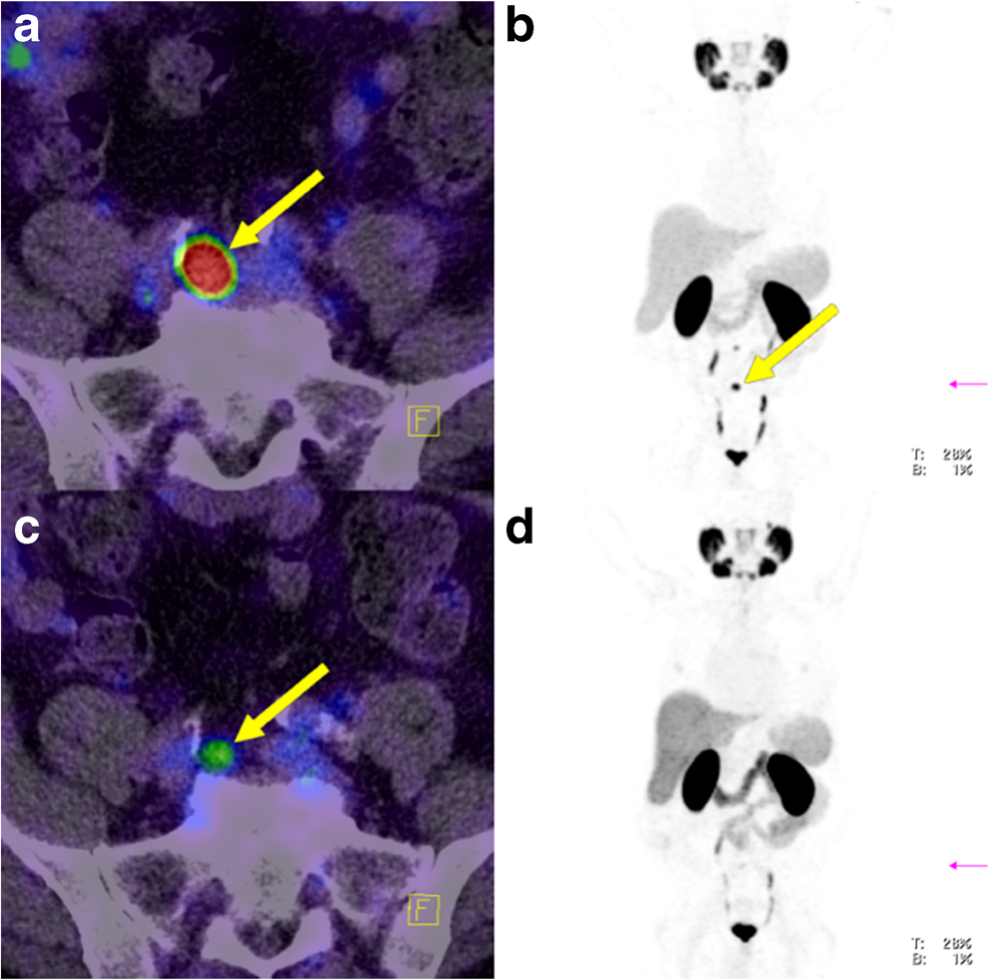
Lymph node metastasis in an example patient (patient 9; *yellow arrows*) clearly visible on PET-1 without ADT (a, b) which became significantly less visible on PET-2 with a complete PSA response to ADT (c, d). a Fused PET and CT image without ADT. b Maximum intensity projection image of the PET data without ADT. c Fused PET and CT image during ongoing ADT. d Maximum intensity projection image of the PET data during ongoing ADT
